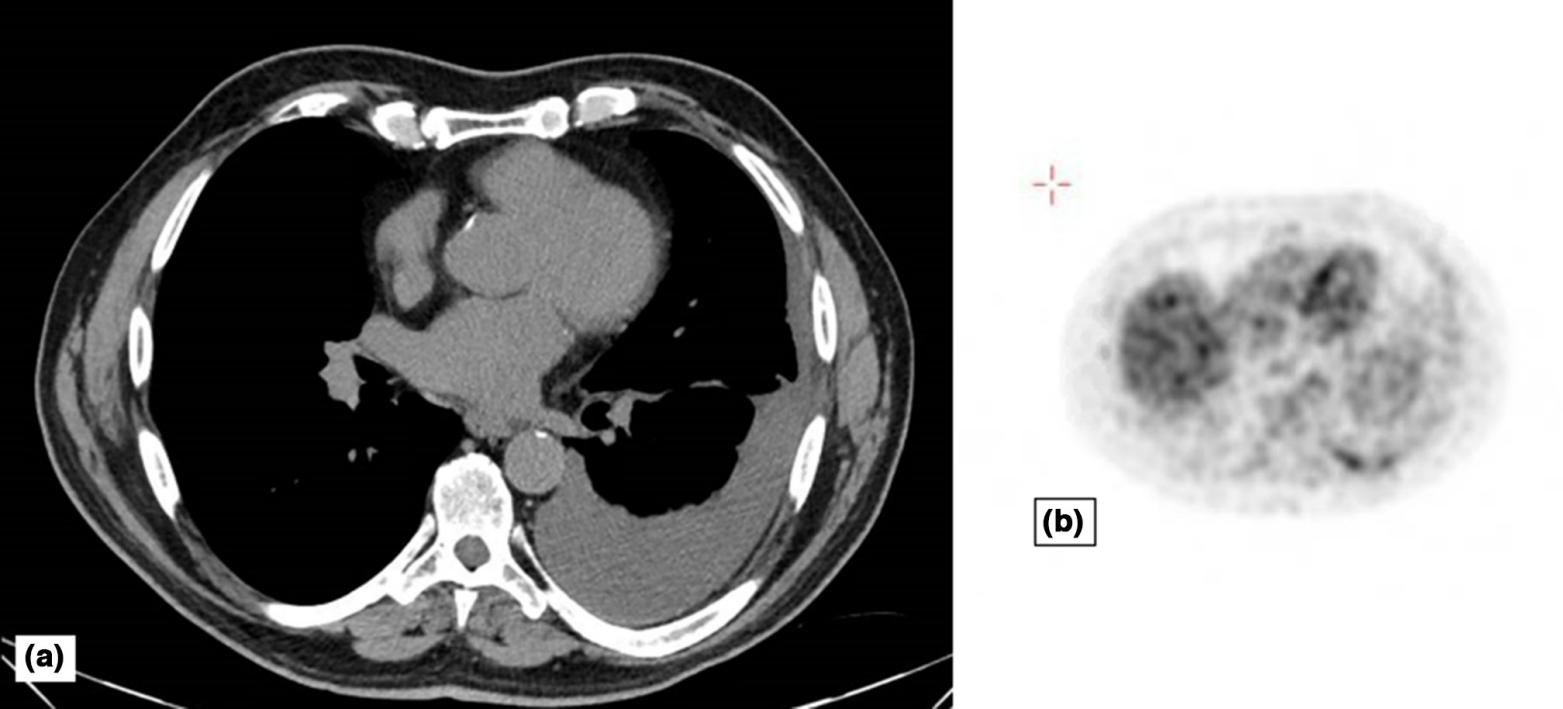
FDG-PET/CT obtained on patient presentation. (a) Left medium-sized pleural effusion. (b) FDG uptake in the left basal dorsal pleura.
